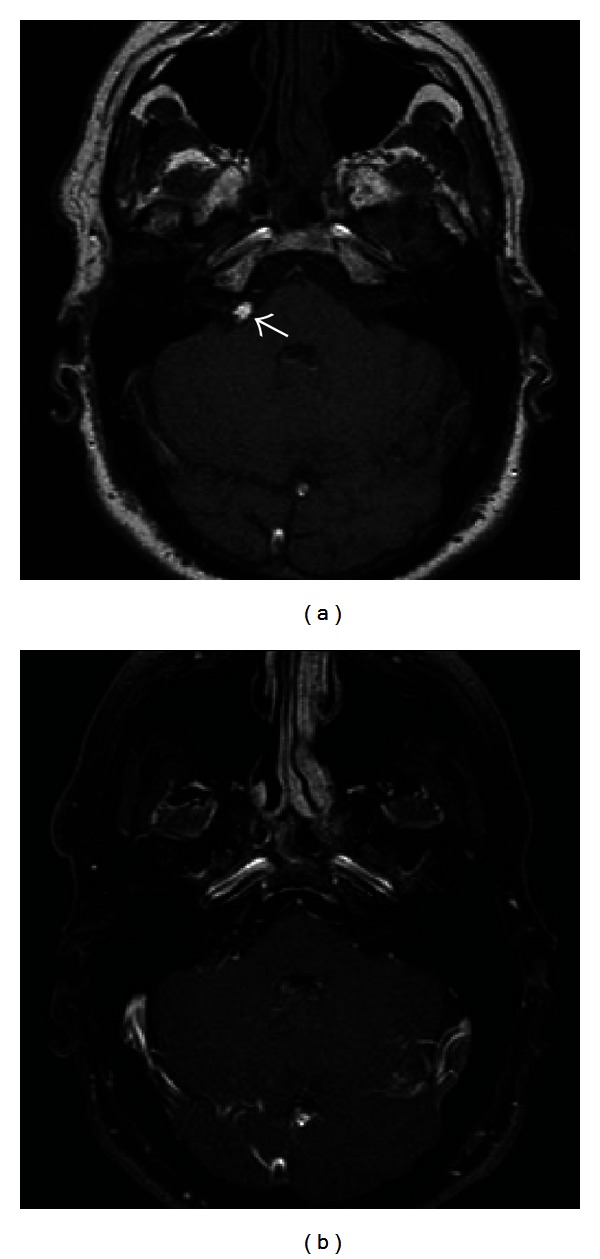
CPA Lipoma. Noncontrast T1-weighted image (a) demonstrates a globular hyperintense lesion in the right CPA (white arrow). (b) Postcontrast fat-saturated T1-weighted MR image shows signal dropout indicating that this lesion is composed of fat. There is no associated enhancement of this lesion.
